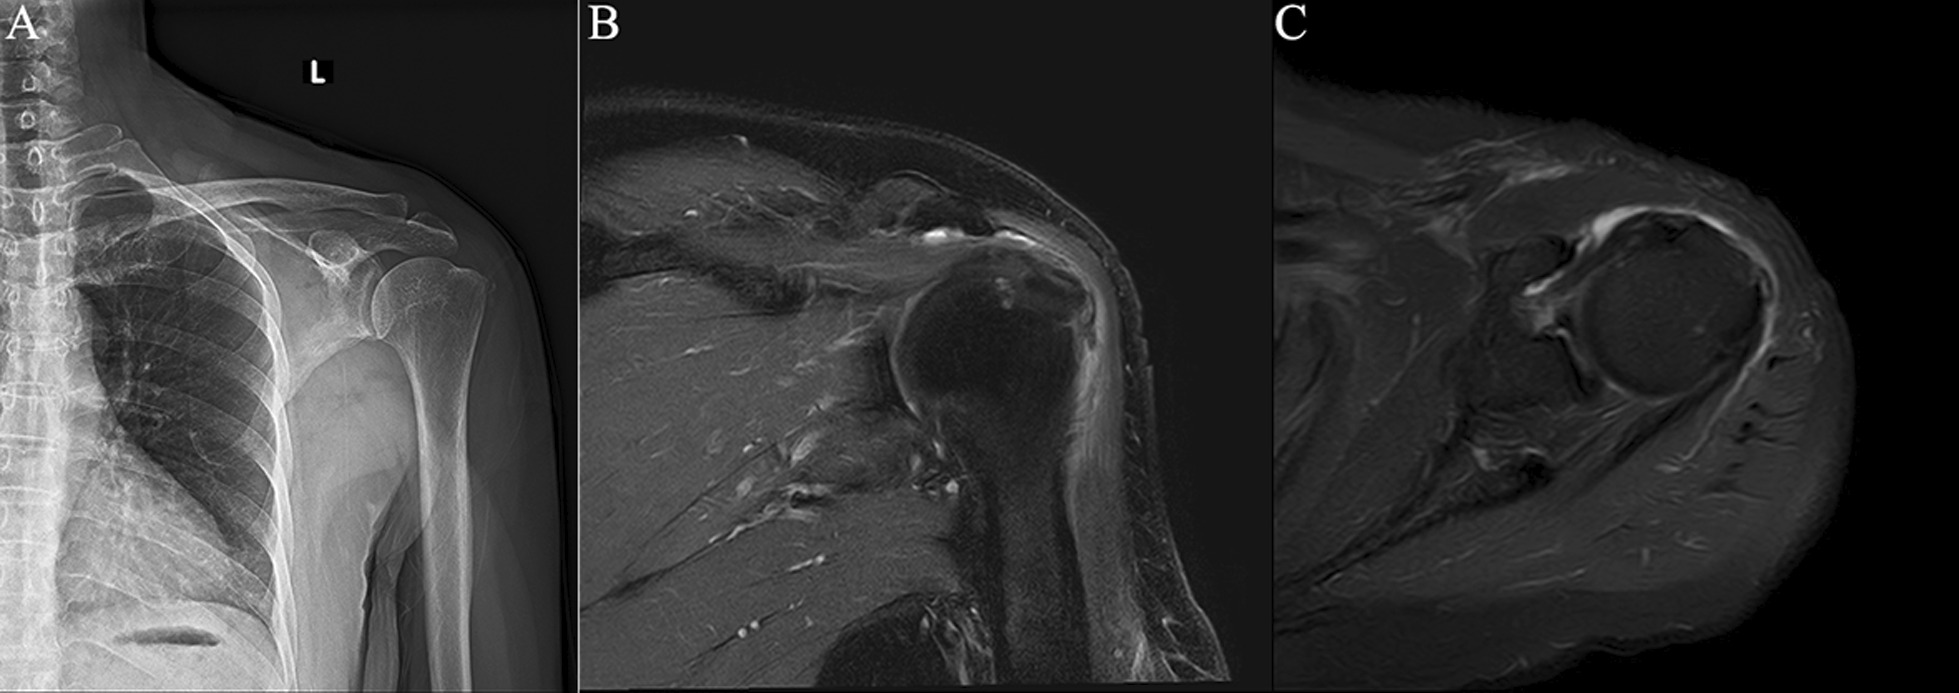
Diffuse tendinitis of the supraspinatus tendon combined with calcific tendinitis of the subspinatus at attachment. *Note* Female, 68 years old, with shoulder periarthritis. A calcifications can be found around the greater tuberosity of humerus on the X-ray film of the left shoulder joint; B T2-SPAIR oblique coronal position, C 2-SPAIR axial position: signs of diffuse mild edema can be observed in the supraspinatus tendon, combined with low signal calcification at attachment of the infraspinatus muscle of the greater tuberosity
